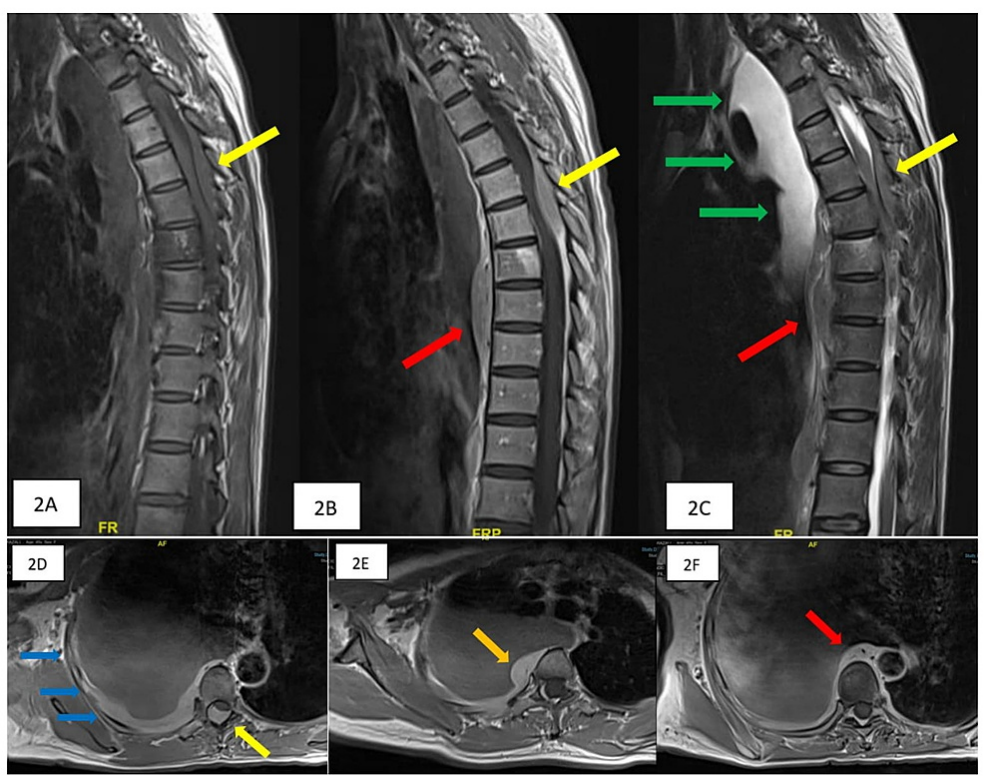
Magnetic resonance images of the thoracic spine. The sagittal plane (A-C) shows an intraspinal extradural lesion extending from T4 to T7 (yellow arrow), measuring about 6 cm in length. It is slightly hyperintense to the spinal cord on T1WI (A) and T2WI (C) and shows marked enhancement on T1 post-contrast images (B). (D-F) T1 axial post-contrast images. Abnormal signal intensity and contrast enhancement are also seen at the T7 vertebral body (A-C). The extradural mass has compressed and displaced the thecal sac left anterolaterally (D) with total obliteration of the subarachnoid space at the level of T6. Homogeneously enhancing prevertebral (red arrow) (A-C, F at the level of T7-T9) and right paravertebral lesions (orange arrow) (E, at the level of T2-T9) are also noted. There is also thickening of the right pleura (blue arrows) (D) with right pleural effusion (green arrows).
